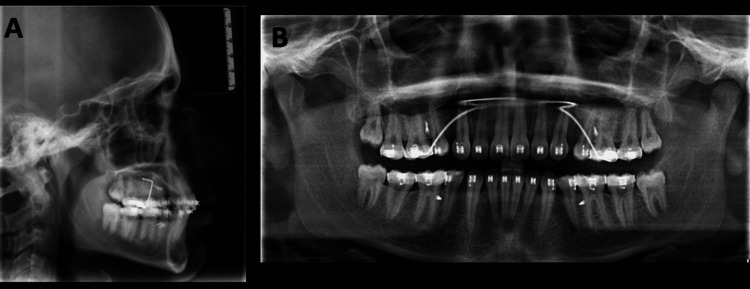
Post-retraction (A) cephalometric radiograph and (B) orthopantomogram
